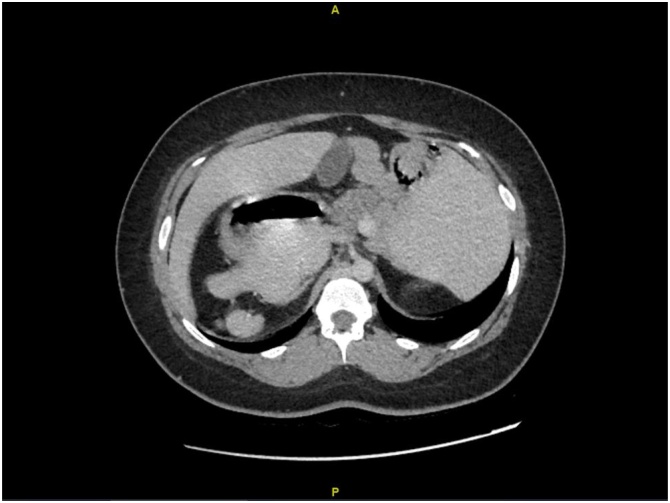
This is the patient’s CT scan in an axial plane, in which you can see a right sided stomach, and you can see the liver’s edges on both the right on left side.
